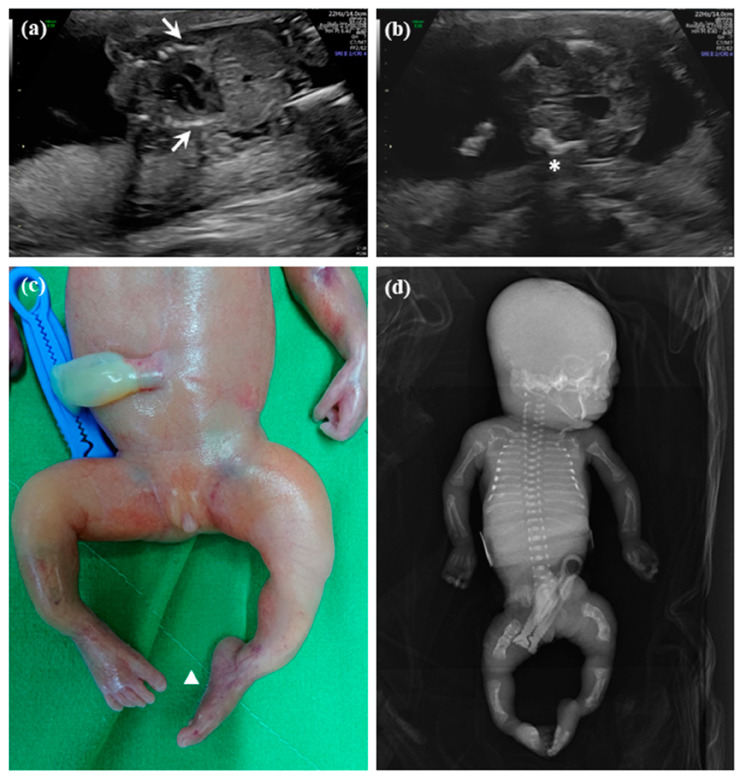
Prenatal ultrasonography of Patient 1 (a female fetus) with osteogenesis imperfecta type IX (OI IX), a kind of skeletal dysplasia (SD), in Family 1 at gestation age (GA) = 19 weeks and 5 days showed (a) bell-shape chest (arrow) and (b) bowing of femur (star). The appearance of the abortus showed (c) talipes equinovarus (triangle). Postmortem whole-body x-film showed (d) thinning of ribs, and bowing of femurs, tibias, and fibulas.
